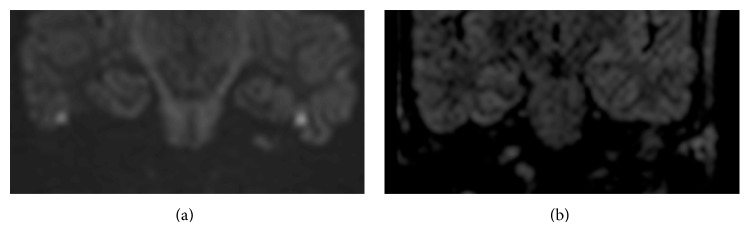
Images of left middle ear cavity in patient with clinical suspicion of primary cholesteatoma: example of SSh SE-EPI DWI false positive (a) compared to MSh TSE DWI true negative (b).
